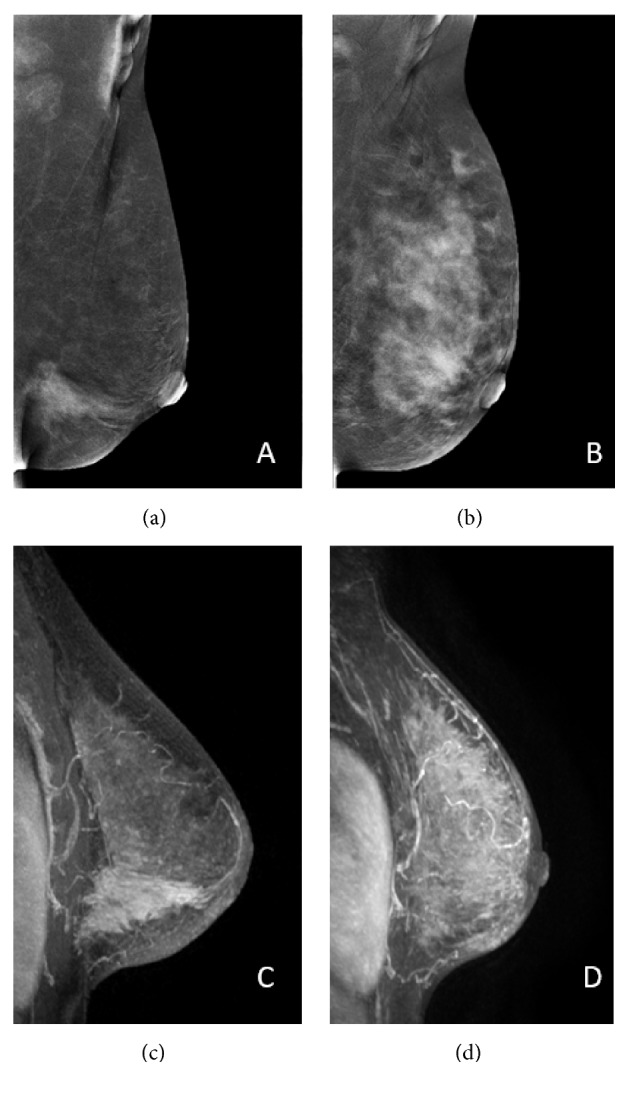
34-year-old woman. Invasive ductal carcinoma with progressive disease. Segmental non-mass enhancement is detected in the lower inner quadrant of the left breast on contrast-enhanced mammography (a) and magnetic resonance imaging (c) before NAC. After NAC, a diffuse non-mass enhancement is detected on CEM (b) and MRI (d).
